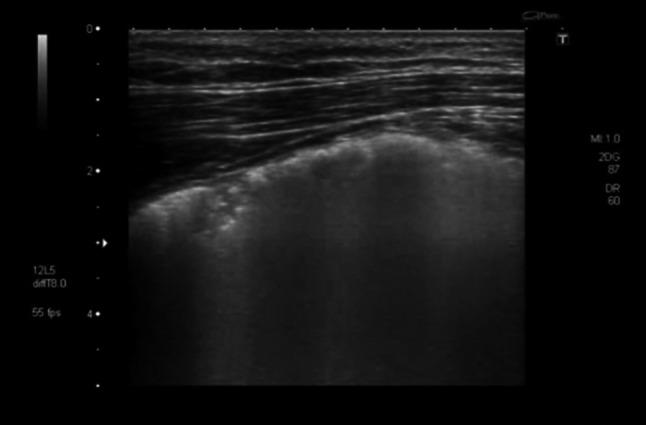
Transthoracic ultrasonography of the pleura shows an interstitial pattern with multiple B-lines. Additionally, small subpleural consolidations are present
